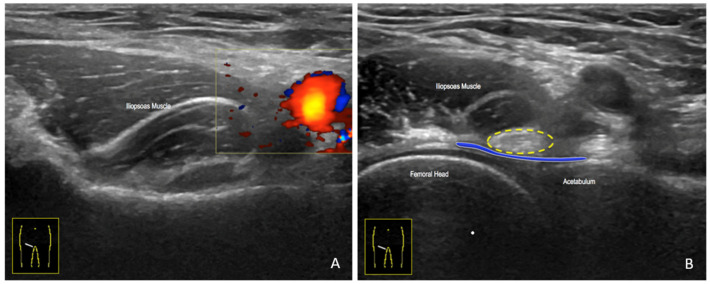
Probe position over the anterior hip at different transverse planes for the evaluation of the iliopsoas muscle, tendon and bursa. (A) Cranial US axial scan showing the muscle bellies of the iliopsoas, coursing in the lateral pelvis over the iliac bone, and the femoral neurovascular bundle with the femoral artery imaged on color-Doppler mode. (B) Caudal US axial scan which shows the iliopsoas muscle belly with its tendon in the typical eccentric position (dashed oval). This is the level to look for the iliopsoas bursa (dark blue), which intervenes between the tendon and the anterior capsule of hip.
